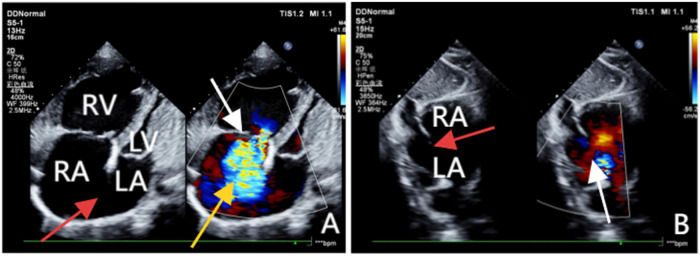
(A) Obtained via left lateral decubitus transthoracic echocardiography, comprises two images. The left panel, a two-dimensional view, clearly demonstrates a loss of continuity in the atrial septum and enlargement of the right atrium and ventricle. The right panel, a Doppler ultrasound, highlights the tricuspid valve (white arrow) with a jet of regurgitant blood flow across it (yellow arrow). (B) Acquired from the subcostal window with the patient in a supine position, clearly reveals the discontinuity in the atrial septum (red arrow) and a left-to-right shunt across the defect (white arrow).
